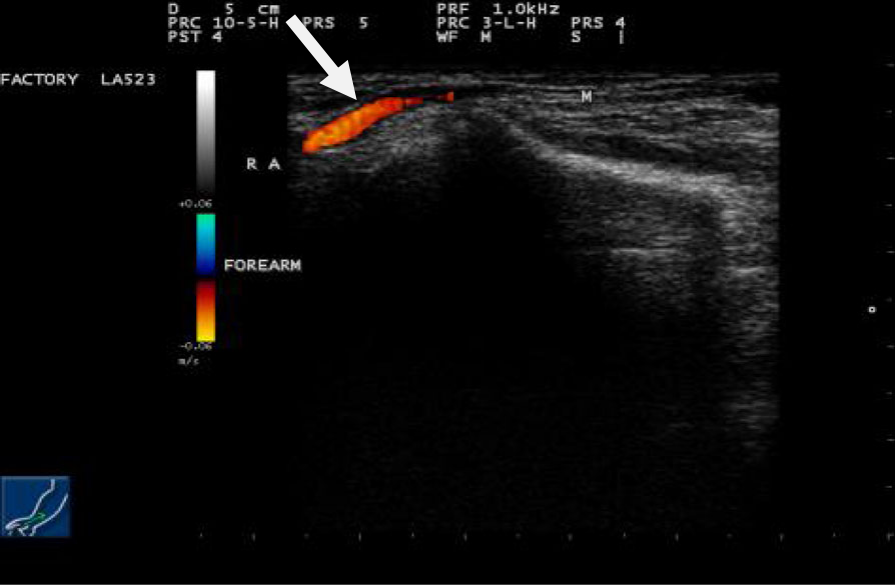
Doppler ultrasonographic image of the right forearm in longitudinal view revealing the muscle mass completely separated from the radial artery (the *arrow* indicates the radial artery)
